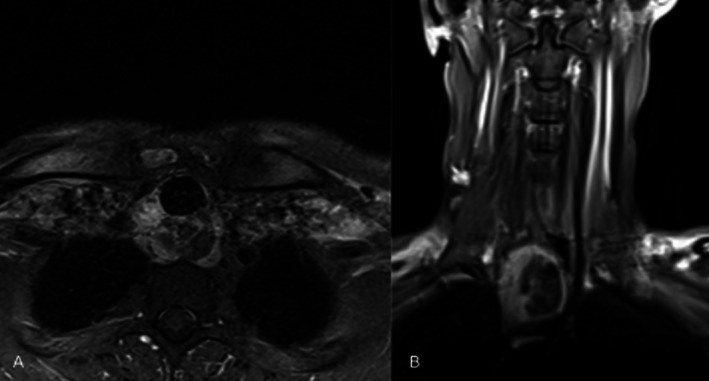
MRI neck and chest. (A) Axial T1 post contrast, fat suppressed MRI shows tumor encasing at least 180 degrees of the posterior tracheal wall, with mass effect onto esophagus causing leftward displacement without clear invasion. (B) Coronal T1 post contrast, fat suppressed MRI shows encapsulated tumor in the maximal craniocaudal direction encompassing the thoracic inlet.
